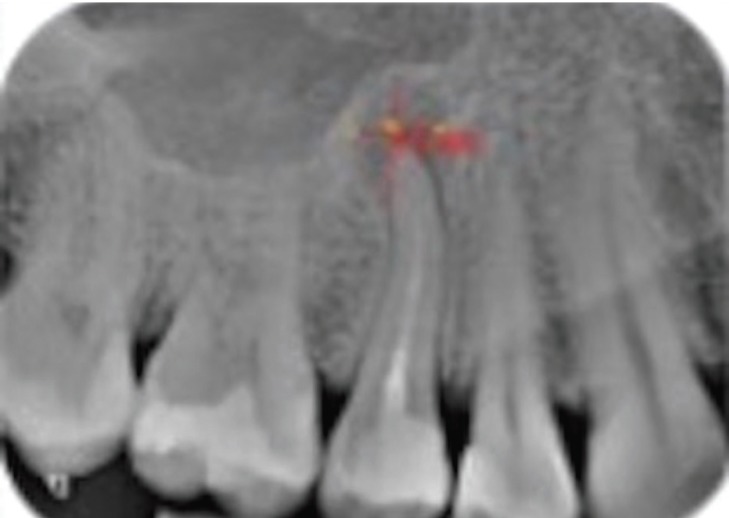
Vertical and horizontal measurements obtained by periapical radiography.
